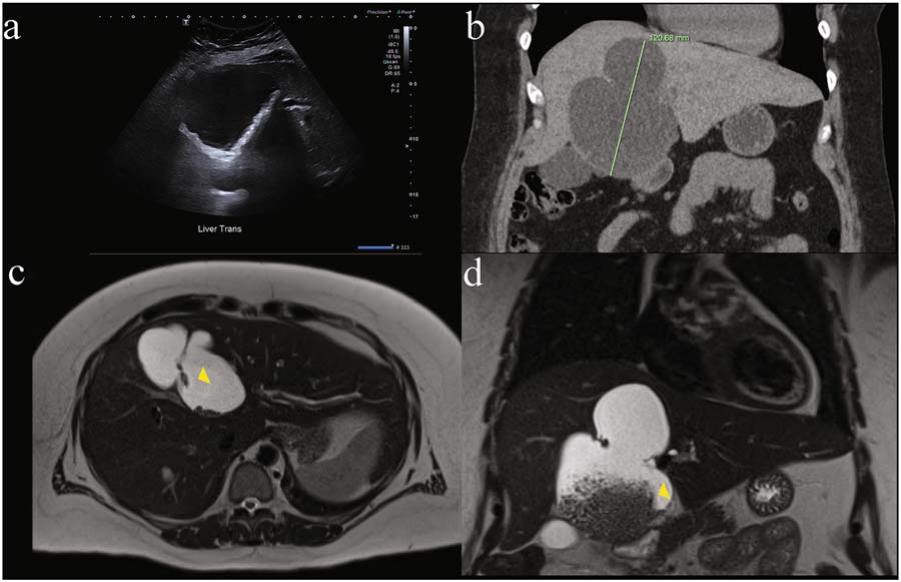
Diagnostic difficulty represented in single patient imaging series of mucinous cystic neoplasm of the liver (MCN-L). There is wide variation between modalities in same patient: (a) ultrasound of liver demonstrating internal matrix echogenicity or debris, (b) coronal contrast enhanced CT showing non-enhancing cystic lesion 12.1 cm in largest dimension occupying most of segment IV a and b with internal debris, peripheral calcifications and left hepatic lobe biliary tree dilatation reported as concerning for biliary cystadenoma, and magnetic resonance imaging (MRI) T2-weighted. (c) Axial and (d) coronal views showing dependent debris with characteristic ‘hydatid sand’ (yellow arrowhead) appearance and septations concerning for hydatid disease that were later found to be bile stones.
